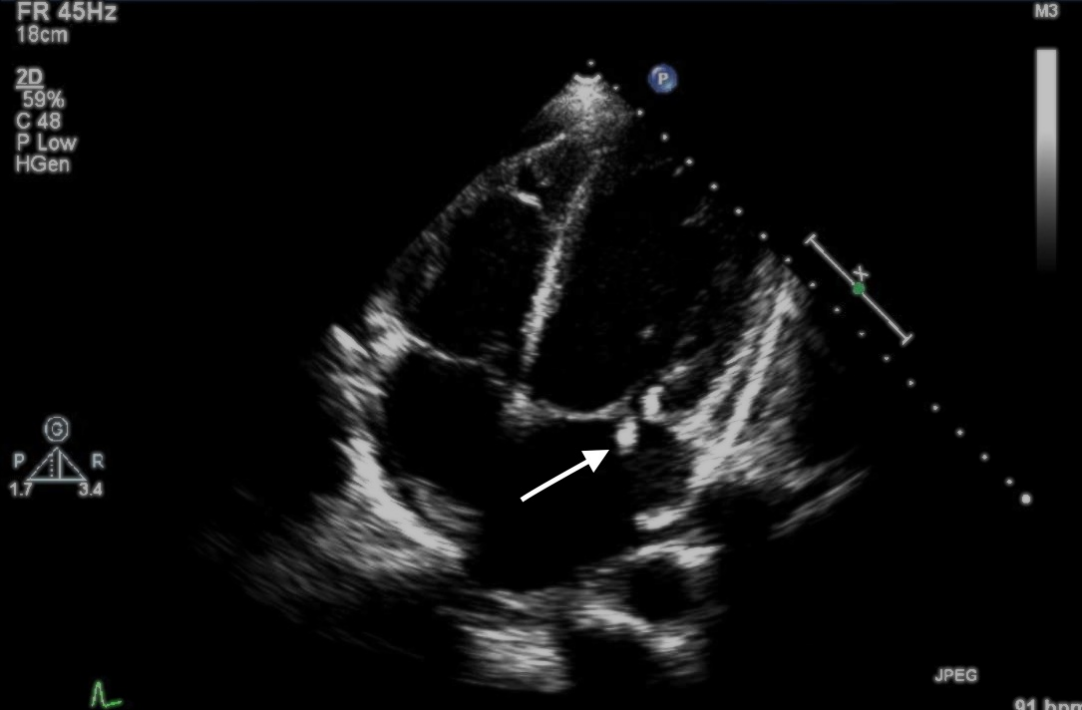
Transthoracic echocardiogram (TTE) showing mobile echodensity (arrow) on the mitral valve leaflet measuring 1.5 x 1.3 cm
